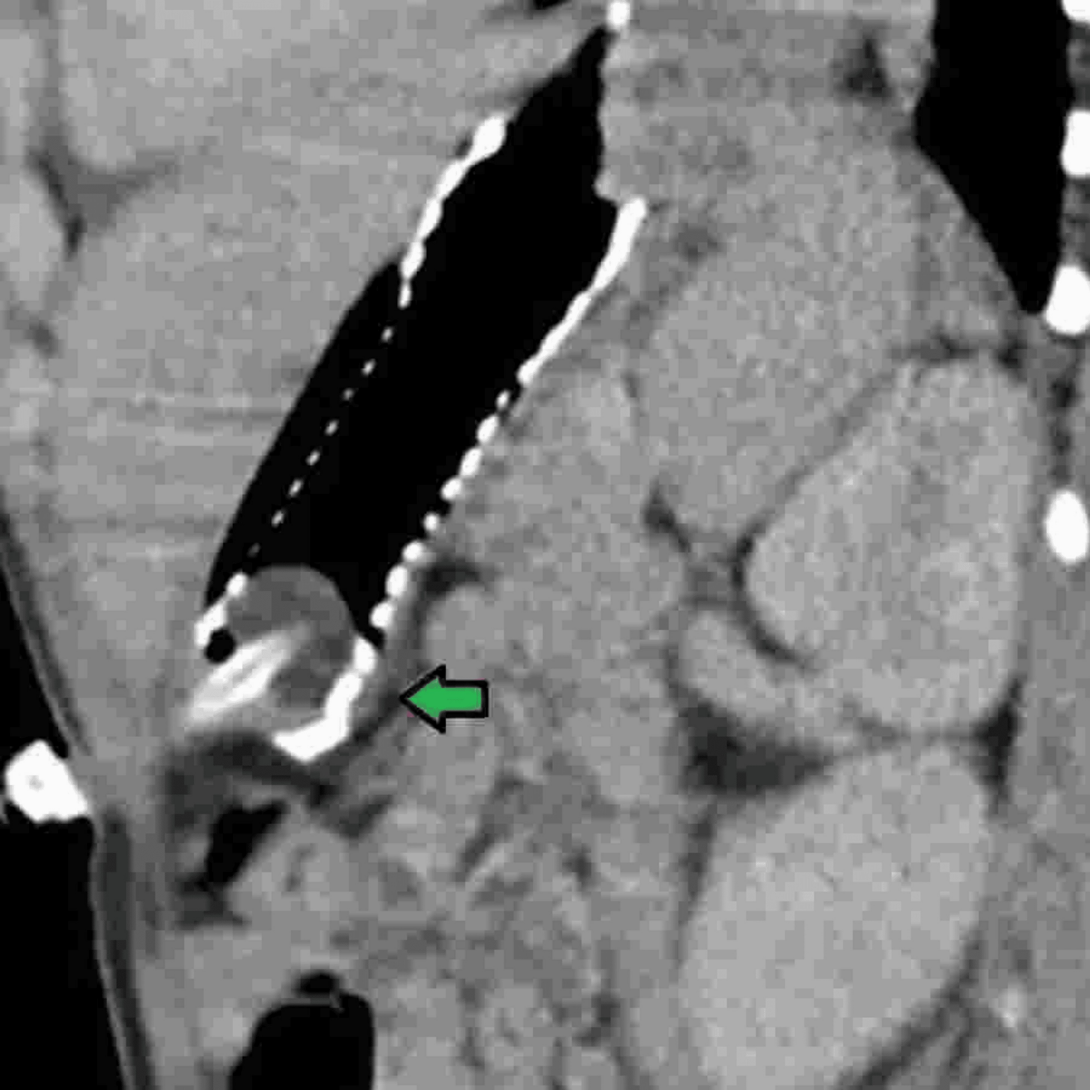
Radiograph image of stent migration to the stomach, with the distal portion wrapped around the gastrostomy balloon (green arrow).
